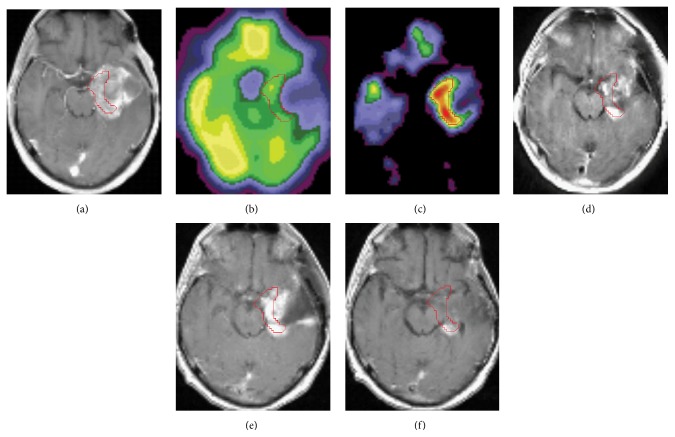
Coregistered PET and MR images from an individual with PNET WHO stage IV (participant #6). Red line delineates the area with the largest increase in AG. ((a)–(c)) MR and PET images at the time of the PET study and 1 day before surgery: (a) T1-weighted image with contrast (Gadolinium) enhancement; (b) CMRGlu PET image; (c) AG PET image. ((d)–(f)) T1-weighted MR images with contrast (Gadolinium) enhancement after partial surgical resection: (d) five days and (e) six weeks after surgery; (f) five months after surgery followed by radiation and chemotherapy.
